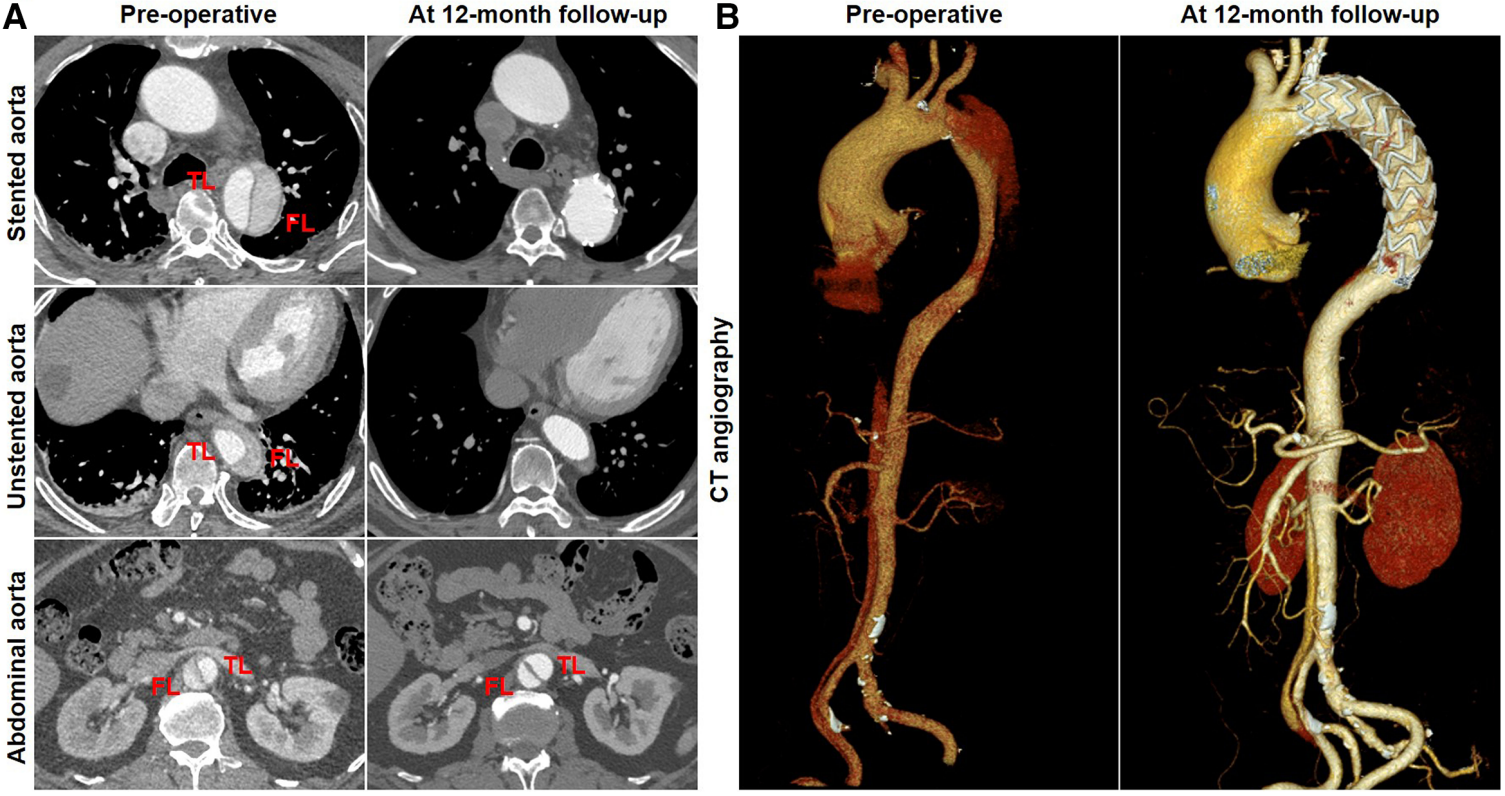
Favorable aortic remodeling after TEVAR with the Castor stent graft. (A) Cross-sectional CT images were obtained from three aortic segments. At the 12-month follow-up, TL enlargement, FL thrombosis and obliteration, and transaortic stabilization were observed over the stented and unstented thoracic aorta; patent FL was stable at the abdominal aorta after TEVAR. (B) Three-dimensional reconstructed CT images exhibited excellent remodeling at the stented and unstented aorta and stable configuration at the abdominal aorta. CT, computed tomography; FL, false lumen; TEVAR, thoracic endovascular aortic repair; TL, true lumen.
